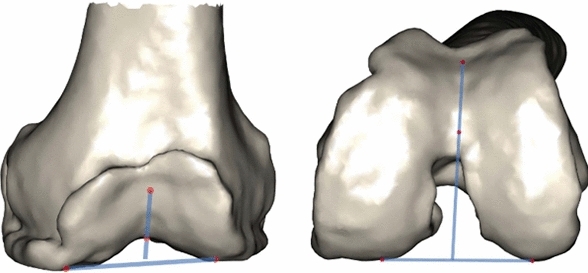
PFJ Radiographic parameters; Trochlea Angle to Distal Femoral Angle (TA-DFA) and Trochlea Angle to Posterior Condylar (TA-PCA)
